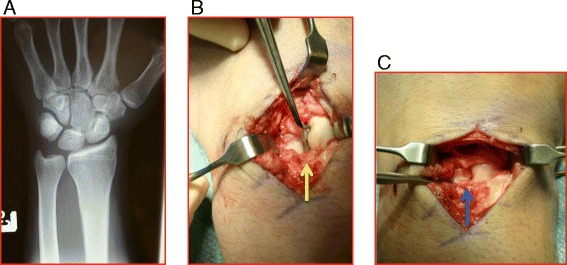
a PA radiograph showing a flexed scaphoid (*signet ring sign*). b Intraoperative finding of a complete SL interosseous ligament tear with the tip of the probe on the scaphoid (*yellow arrow*). c Open reduction of the SL interval (*blue arrow*) prior to ligament repair
